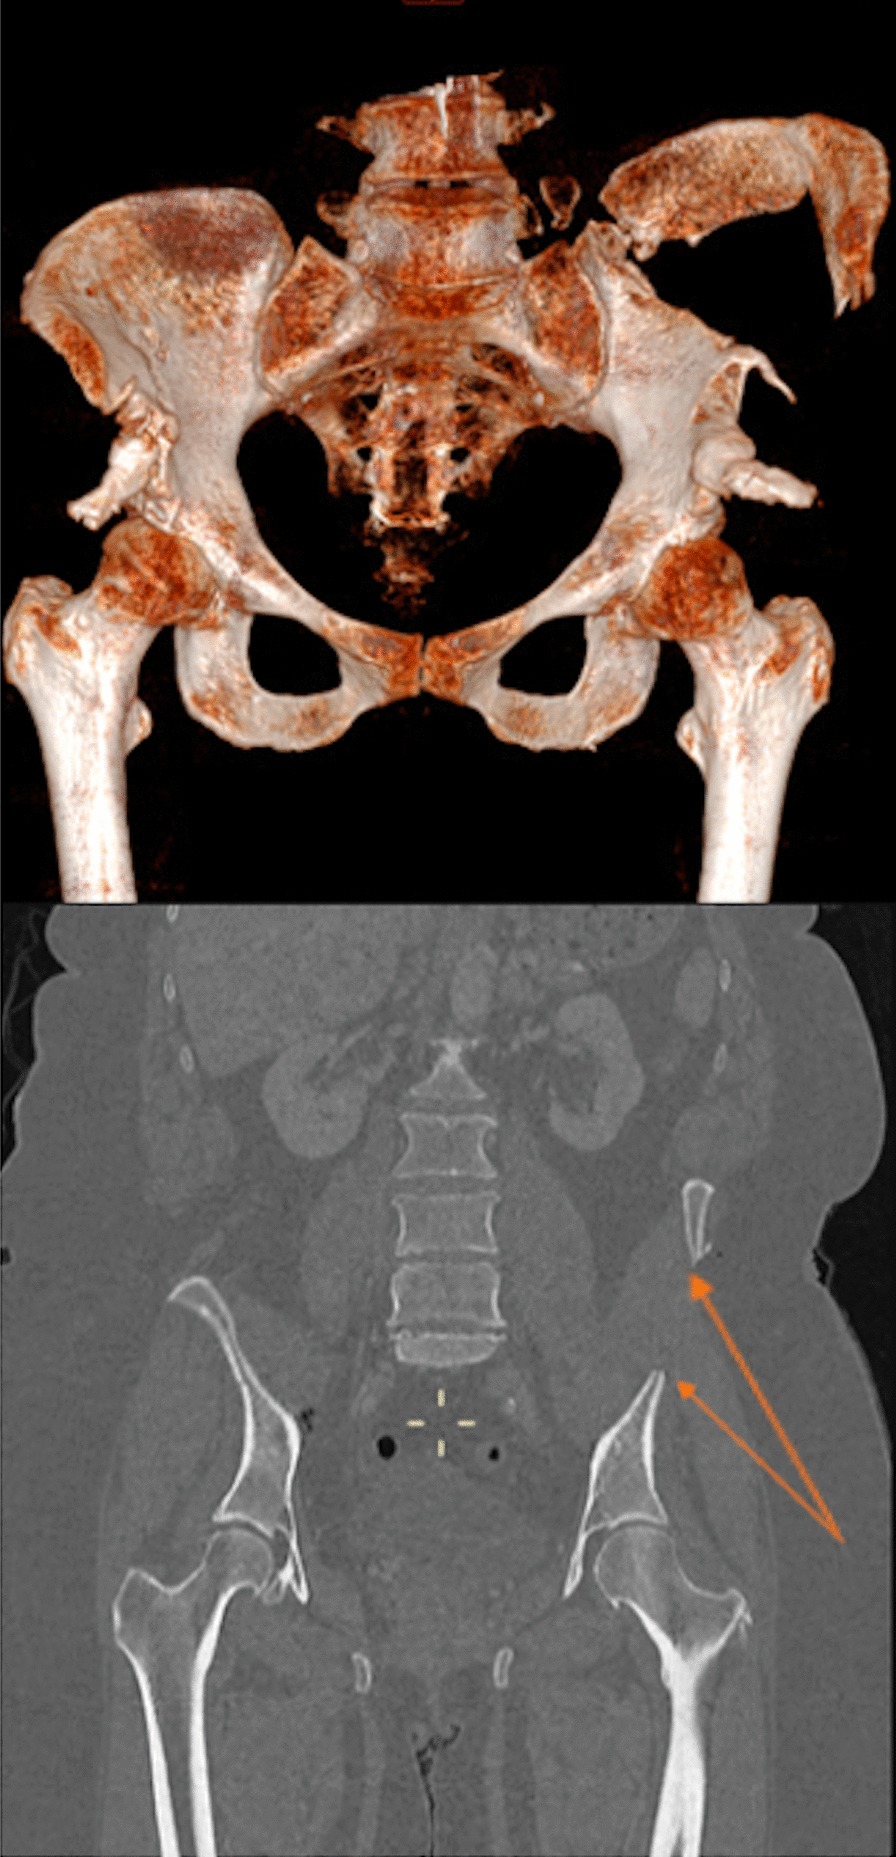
Abdominal CT scan: fracture of the left iliac crest (arrow)
